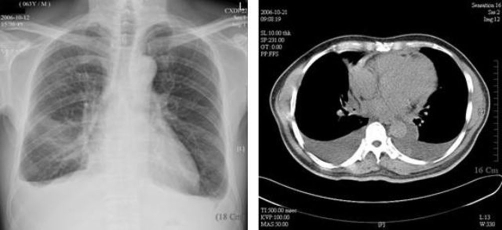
Image studies of the patient. (A) A chest X-ray; (B) a CT scan of the thorax showing thickened pericardium with areas of calcification, and bilateral pleural effusions.
